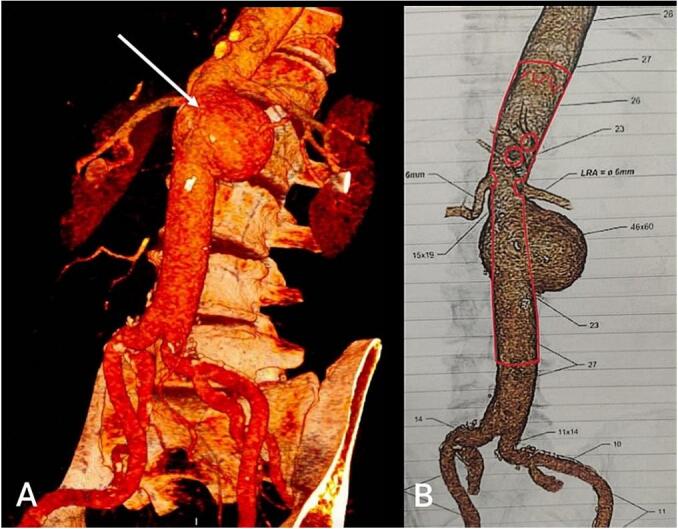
A) CTA showing pseudoaneurysm adjacent to the previous proximal anastomosis of aortoaortic bypass graft (case 1). The juxta-renal position and short landing zone (14 mm to the superior mesenteric artery) make the open repair and other endovascular options such as EVAR and CHEVAR not feasible. B) Planning sketch of FEVAR graft used for case 1.
